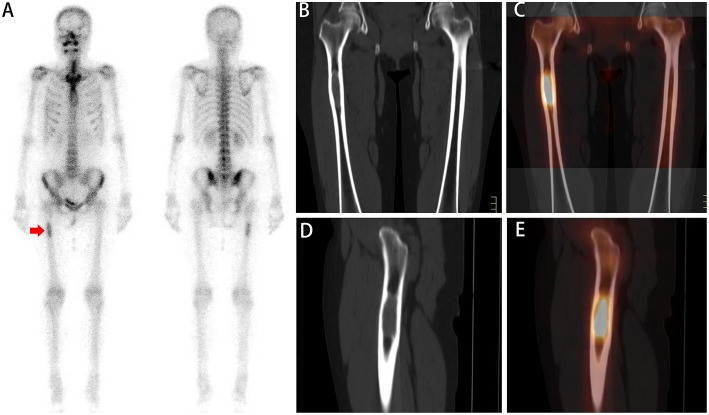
A whole-body scan (A) revealed a focal area of low ^99m^Tc-MDP activity in the right femur (red arrow). No additional areas of abnormal ^99m^Tc-MDP uptake were identified in the remainder of the skeleton. Coronal (B,C) and sagittal (D,E) CT and SPECT/CT imaging showed focally increasing ^99m^Tc-MDP uptake corresponding to lytic changes and soft tissue masses in the diaphysis of the femur. A biopsy was subsequently performed, and pathologic analysis confirmed the diagnosis of bone metastasis.
